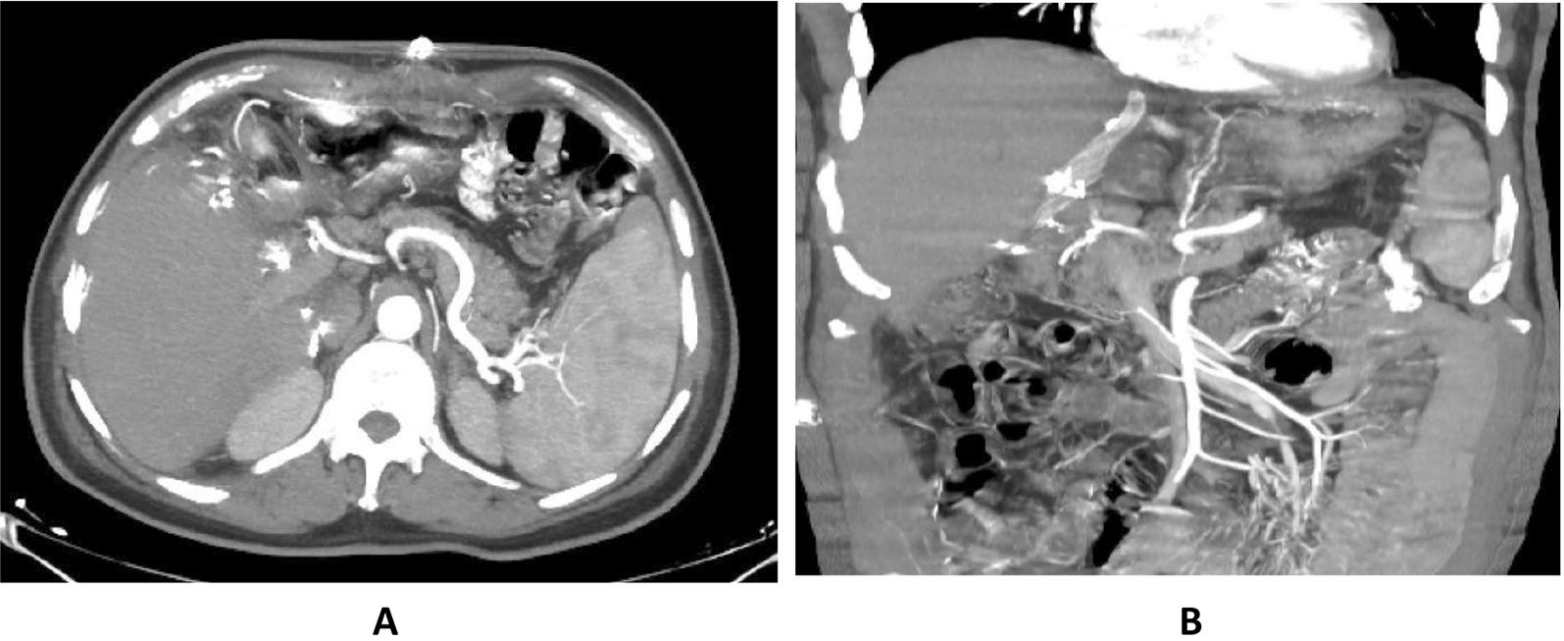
A Axial contrast-enhanced CT images (Mini MIP) showing markedly attenuated caliber of the hepatic artery. B Coronal CT images (Mini MIP); the hepatic artery cannot be traced at the porta hepatis
